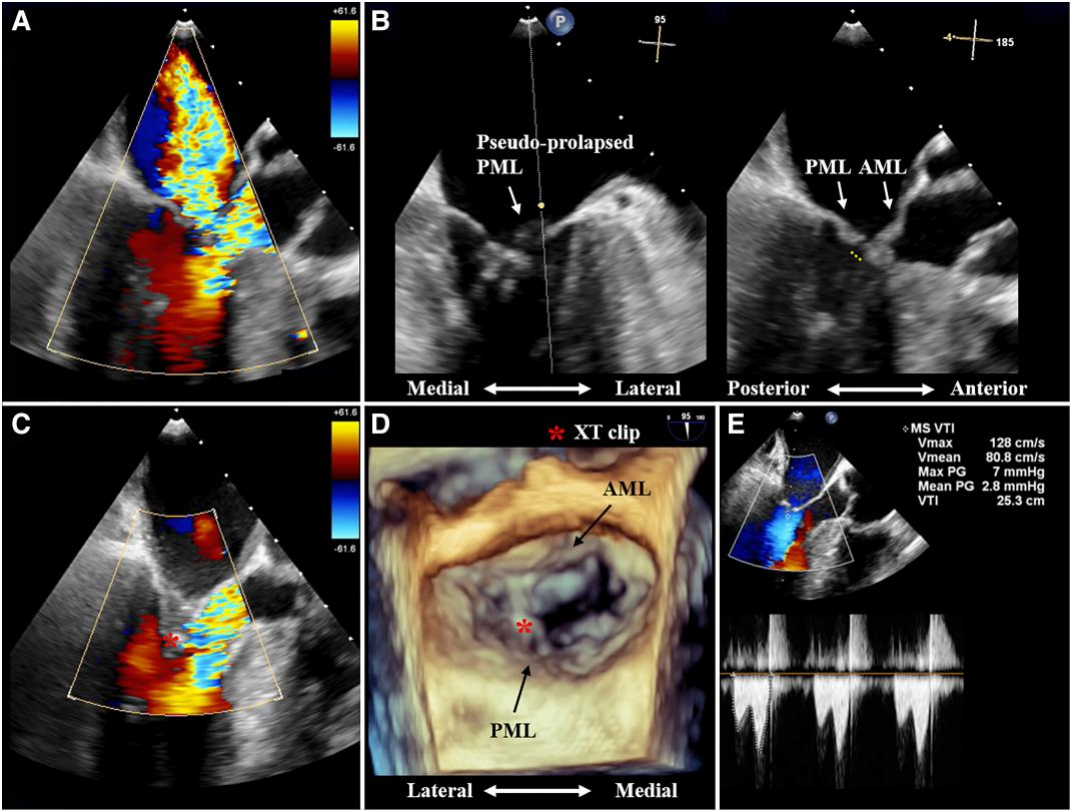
Transoesophageal echocardiography evaluation during MitraClip therapy. (*A* and *B*) Transoesophageal echocardiography shows that the massive systolic anterior motion–related mitral regurgitation is caused by a newly developed large mitral leaflet coaptation gap with a pseudo-prolapse of the posterior mitral leaflet. The white line denotes the coaptation gap. (*C–E*) Transoesophageal echocardiography and a three-dimensional en face view show the successful systolic anterior motion–related mitral regurgitation reduction by an XT clip implantation (asterisks) within an acceptable degree of the mean transmitral valve pressure gradient.
